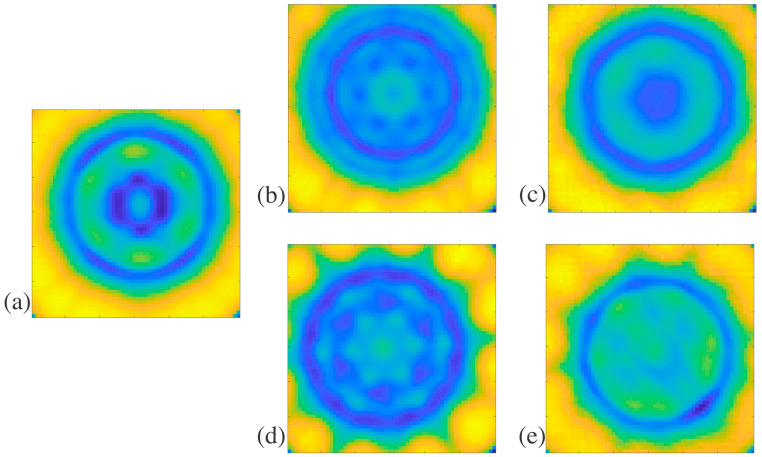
Structure C THz CT slice reconstructions in MATLAB: (a) no post-cure slices without defects, (b) thermal post-cure slice without defects, (c) thermal post-cure slice with blockages, (d) UV post-cure slice without defects, and (e) UV post-cure slice with blockages.
